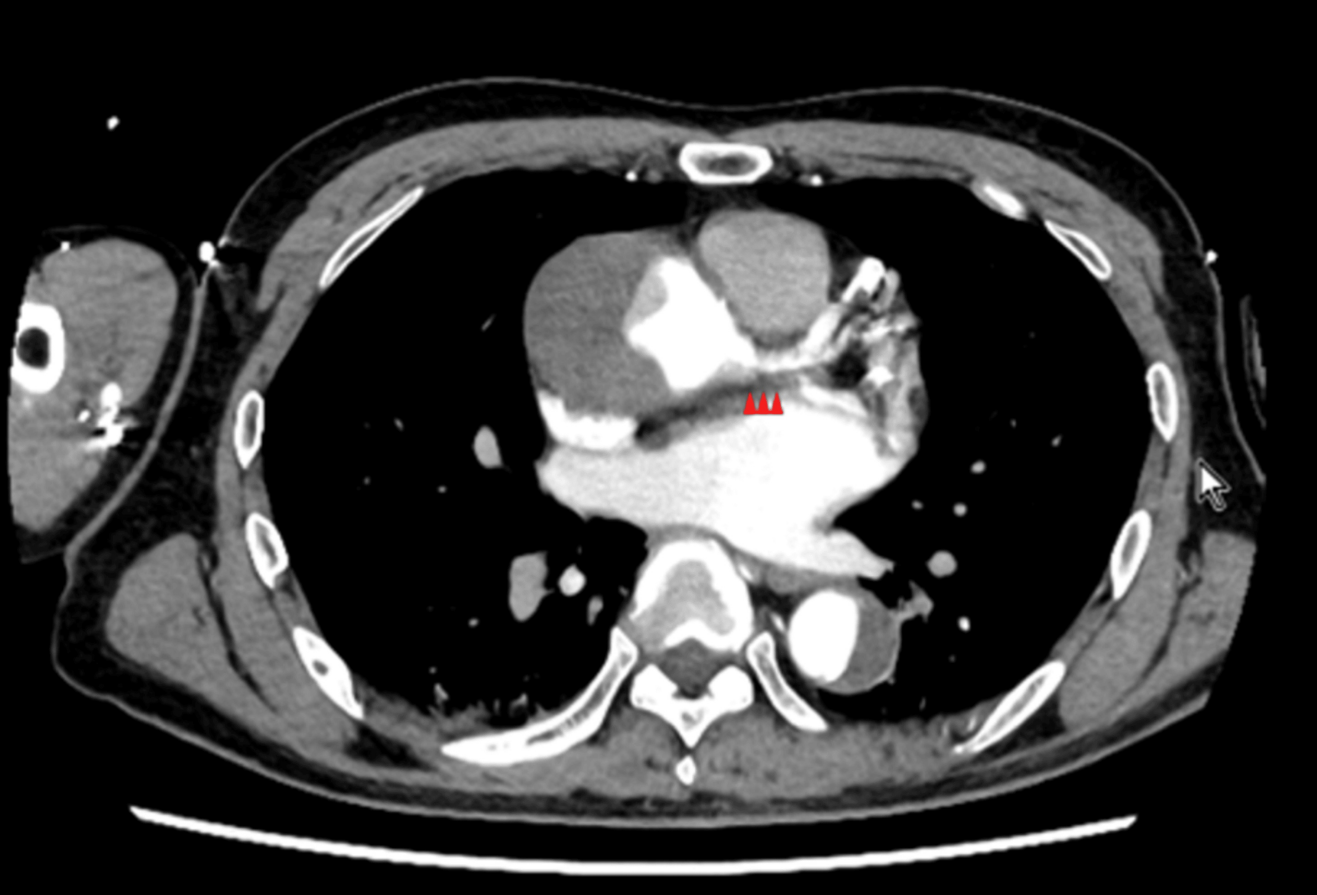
Contrast-enhanced CT demonstrating the presence of thrombotic occlusion in acute type A aortic dissection. The false lumen compresses the ostium of the left main trunk. Arrowheads indicate the false lumen exerting pressure on the ostium of the LMT. CT, computed tomography; LMT, left main trunk.
